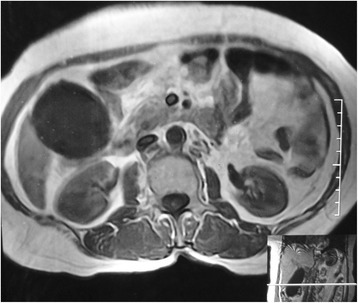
MRI showing mucocele of gall bladder (transverse plane)
